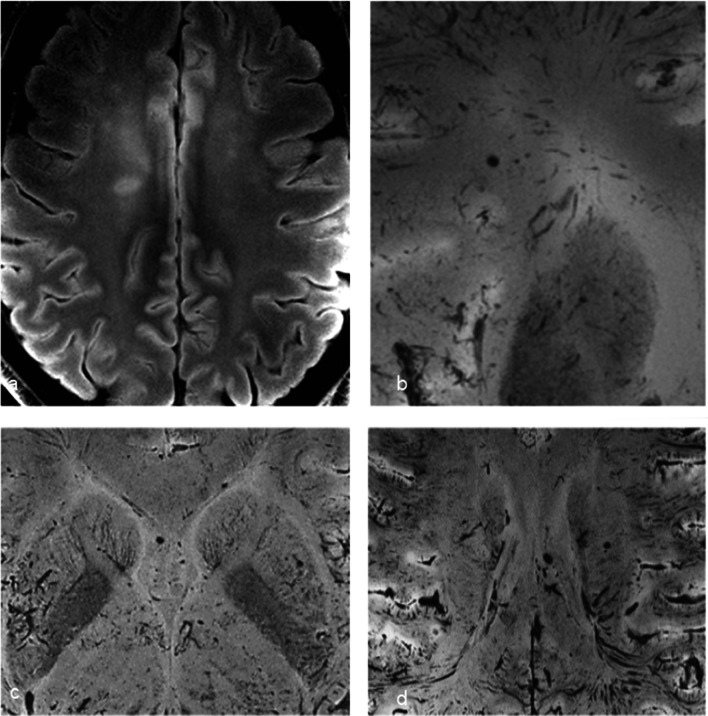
Nonspecific multifocal white matter abnormalities with increased signal on FLAIR (a) and punctate hemorrhagic foci in the right white matter on SWI (b) of patient 5. Patient 7 showed some periventricular punctate hemorrhagic foci on SWI (c, d)
